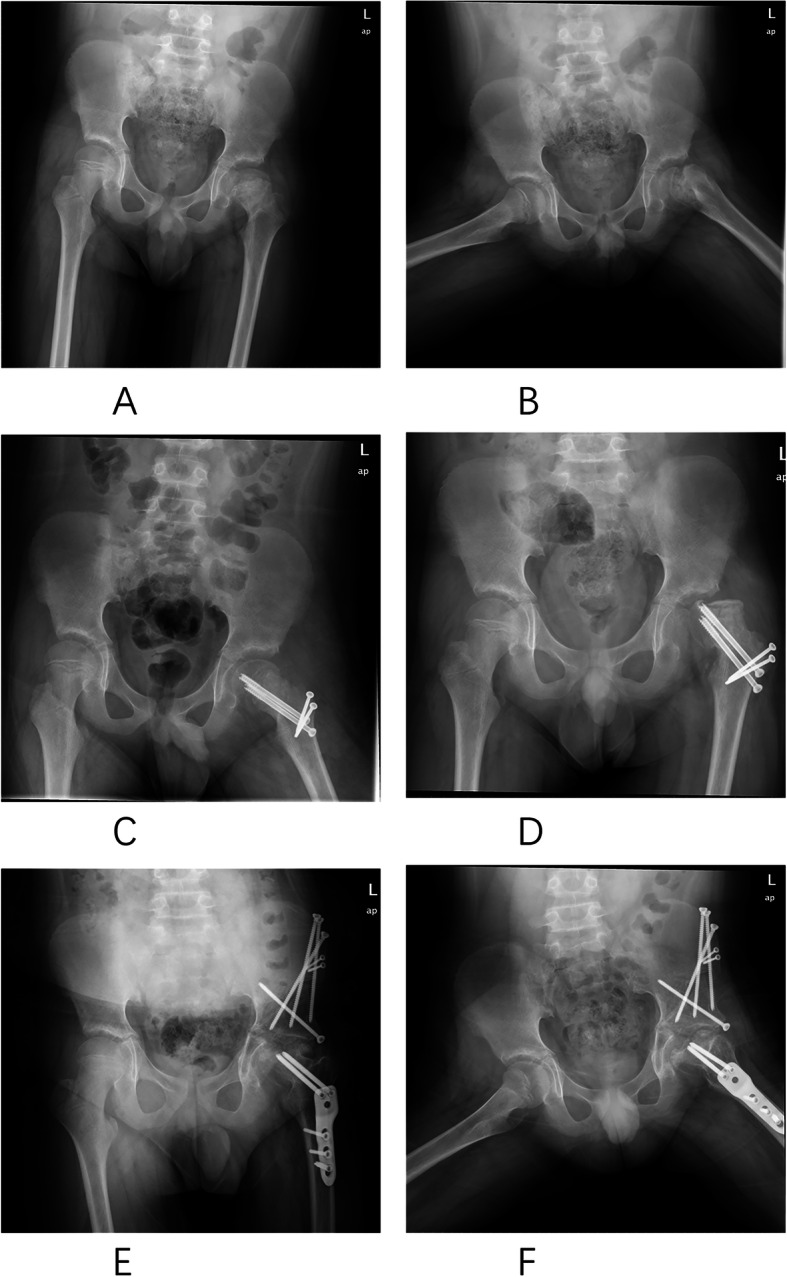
A 10-year-old boy with severe pain and functional disability. a AP and b frog-leg lateral radiographs revealing a severe SCFE of the left hip. c AP showed good restoration of the SCFE after the operation. d Obtained 3 months postoperatively showed implant failure. And finally received periacetabular osteotomy and proximal femur osteotomy surgery, e AP and f frog-leg lateral radiographs. AP indicates anteroposterior
